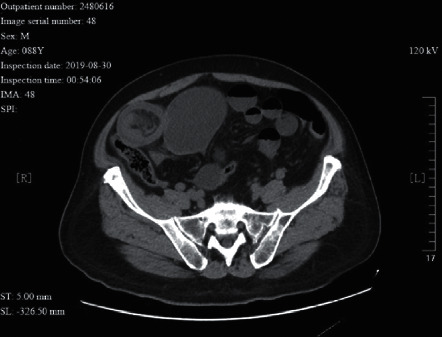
An 88 year-old male patient with intussusception caused by stromal tumor of the small intestine. The CT image shows a target sign. Ischemic necrosis of the intestinal wall was seen during surgery.
